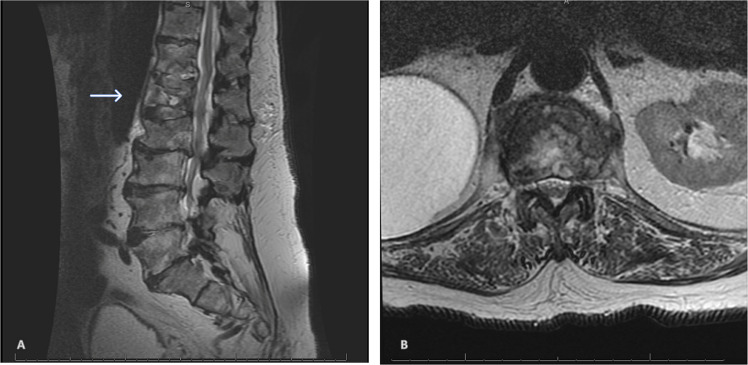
Sagittal (a) and axial (b) T2-weighted MRI with arrow pointing at an L1 vertebral body compression fracture concerning for infection or neoplasm. Markedly abnormal signal within the L1 vertebral body, with significant involvement of T12, and the superior aspect of L2
